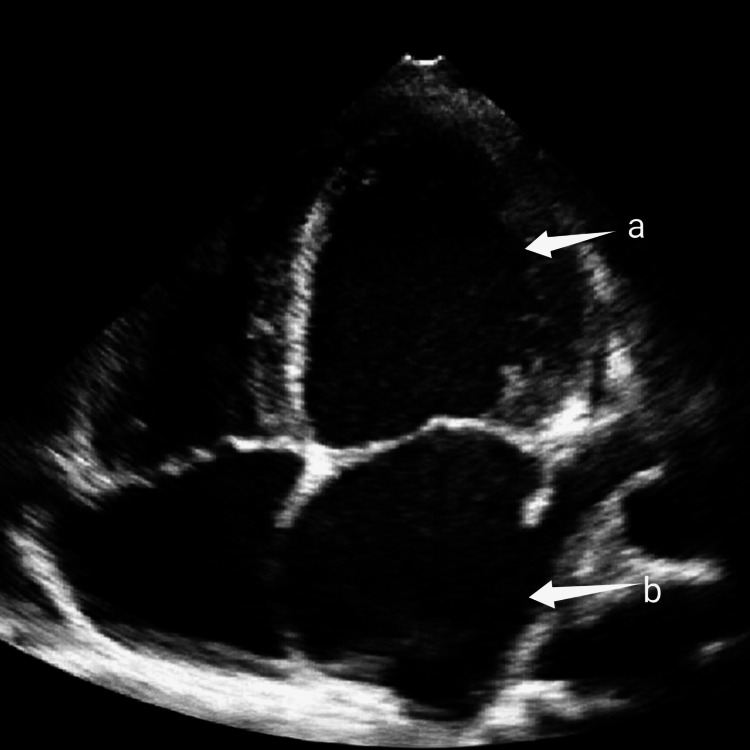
2D echocardiogram apical four-chamber view showing dilated left ventricle (arrow a) and left atrium (arrow b) and eccentric hypertrophy of left ventricle.
